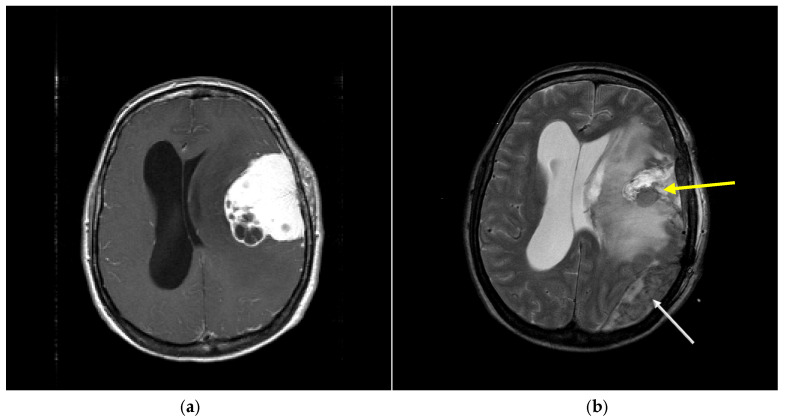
(a) Preoperative axial enhanced T1-weighted MRI revealed a large mass in the left parietal region. (b) Postoperative 24 h MRI showed total tumor resection with the tumor cavity hemorrhage (yellow arrow) as well as epidural hemorrhage at the left occipital area (white arrow). The presence of a second bleeding site makes surgical technique a less favorable risk factor for this hemorrhagic complication.
